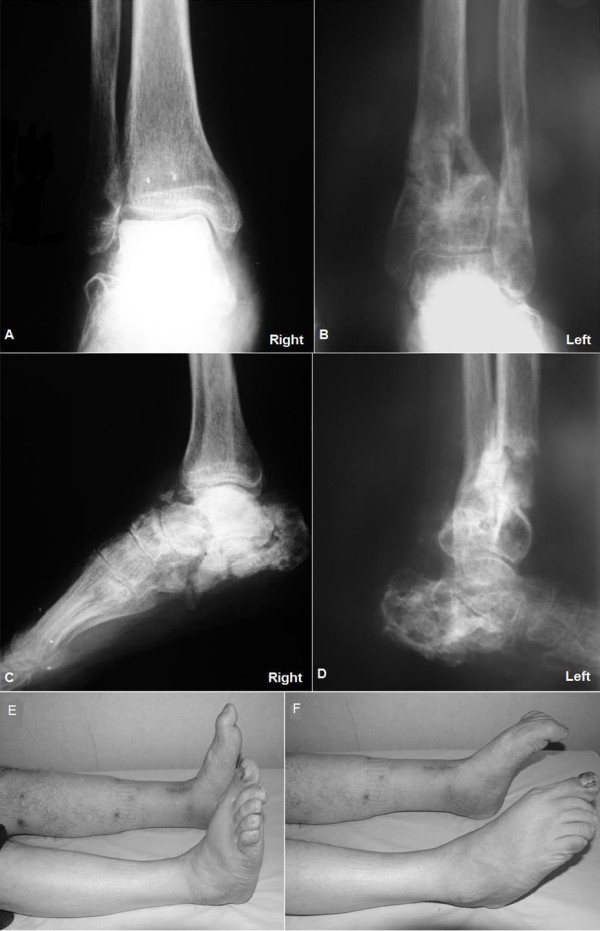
Radiographs (A-D) and clinical pictures (E, F) at the end of the first postoperative year.
